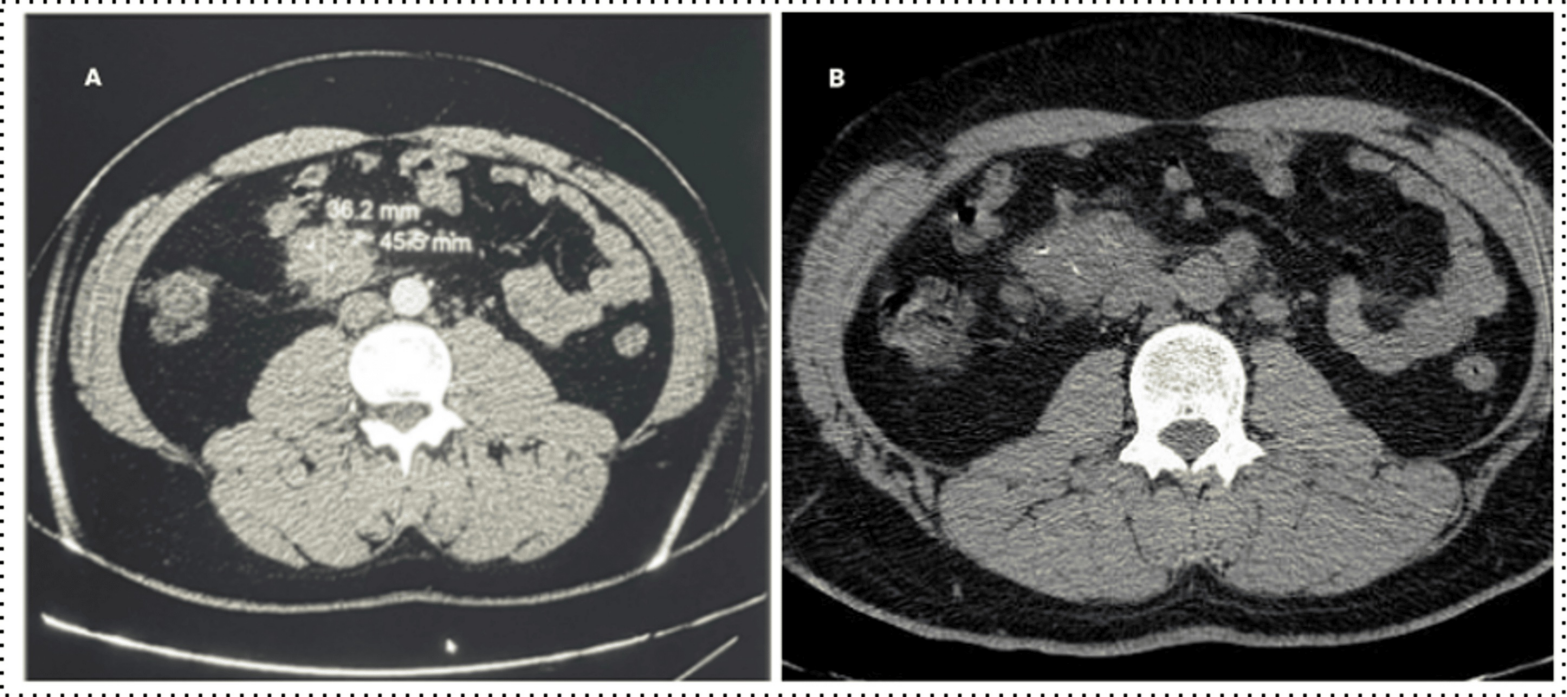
CT images of the patient demonstrating abdominal lymphadenopathy (A) Axial section of a contrast-enhanced abdominal-pelvic CT scan showing a round celiac-mesenteric lymphadenopathy, measuring 36.2 mm and 45.5 mm in diameter with enhancement. (B) Axial section of a non-contrast-enhanced CT scan showing a mesenteric lymphadenopathy with calcifications typical of TB. CT: computed tomography, TB: tuberculosis
